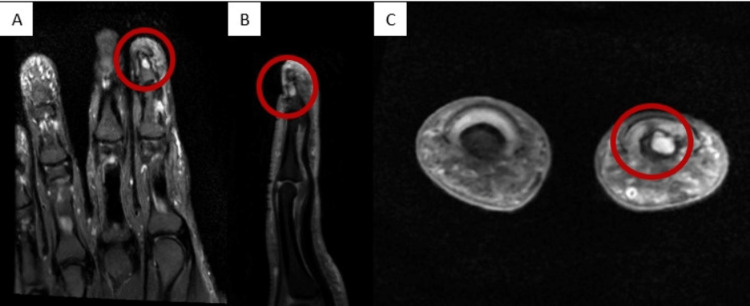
Magnetic resonance imaging: coronal view (A), sagittal view (B), and axial view (C). A well-defined lesion was seen at the distal phalanx of the left index finger within the intramedullary region, measuring 2.7 x 2.1 x 3.2 mm, demonstrating hyperintense signal in T2W (circled with a red marker).
